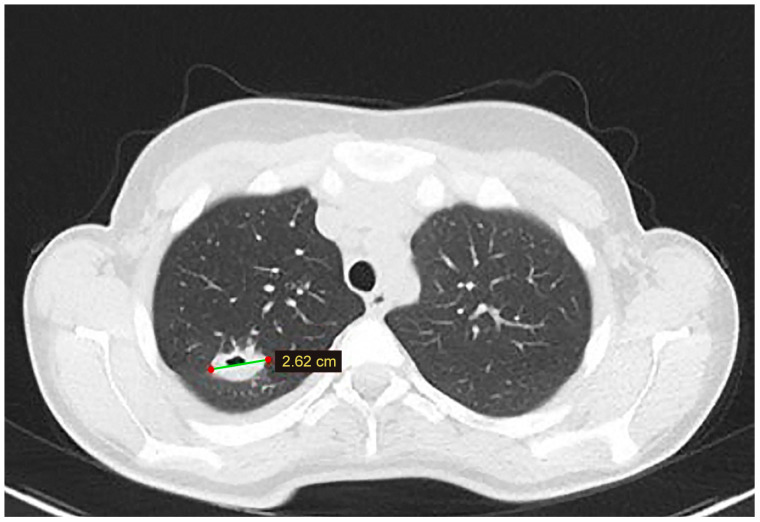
A lung CT scan at the 6-month follow-up—a cystic lesion is discovered in the superior lobe of the right lung.
